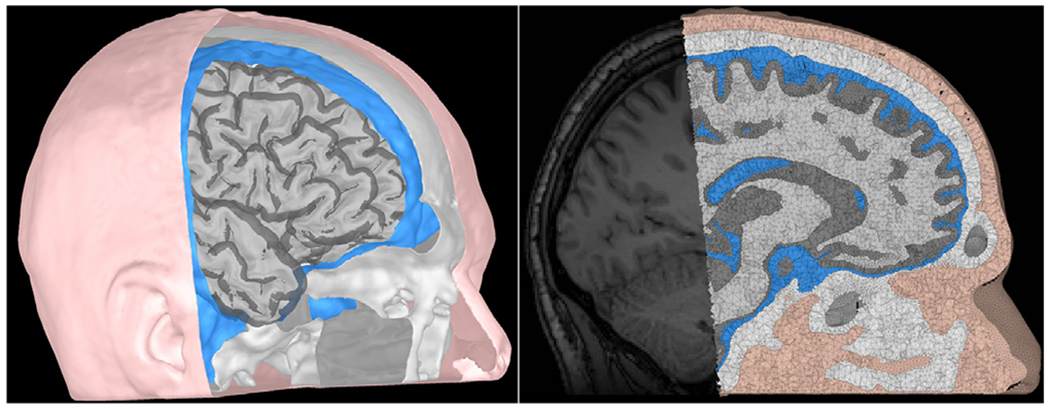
Left: surface head model; tissues from inner to outer: wm, gm, CSF, skull, and scalp. Right: Overlay of the tetrahedral volume mesh on the MRI.
